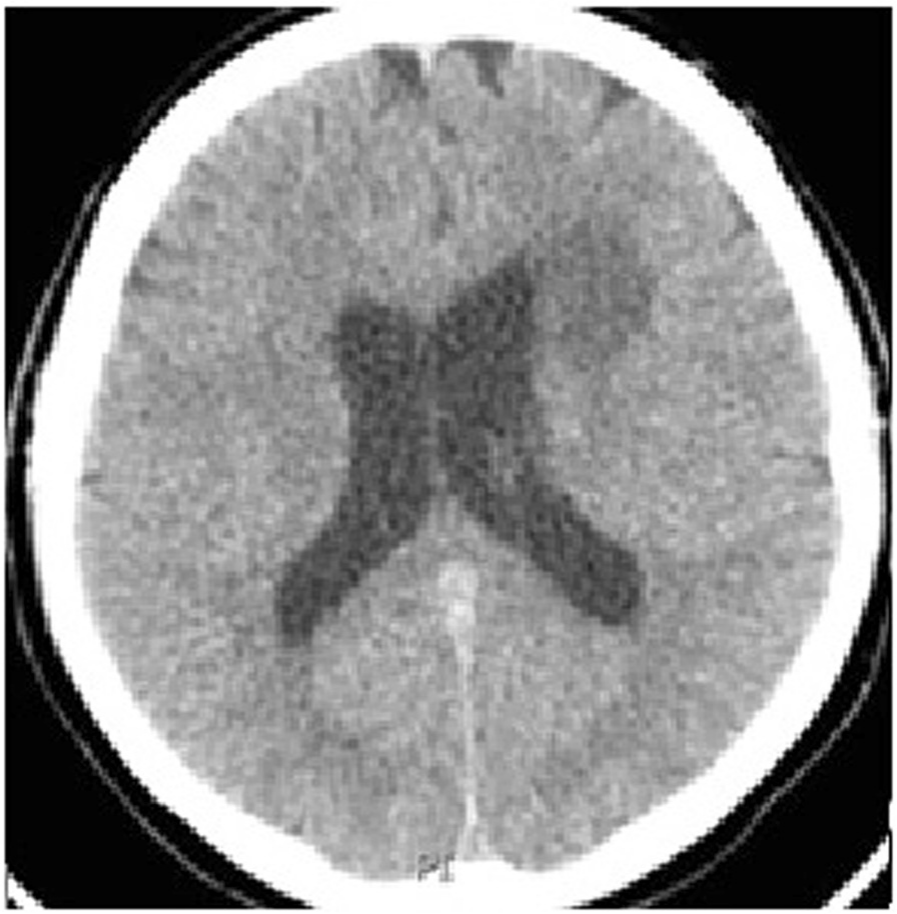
Follow-up computed tomography image (after 1 month) with more than 50% reduction in the size of the mass with minimal brain edema
